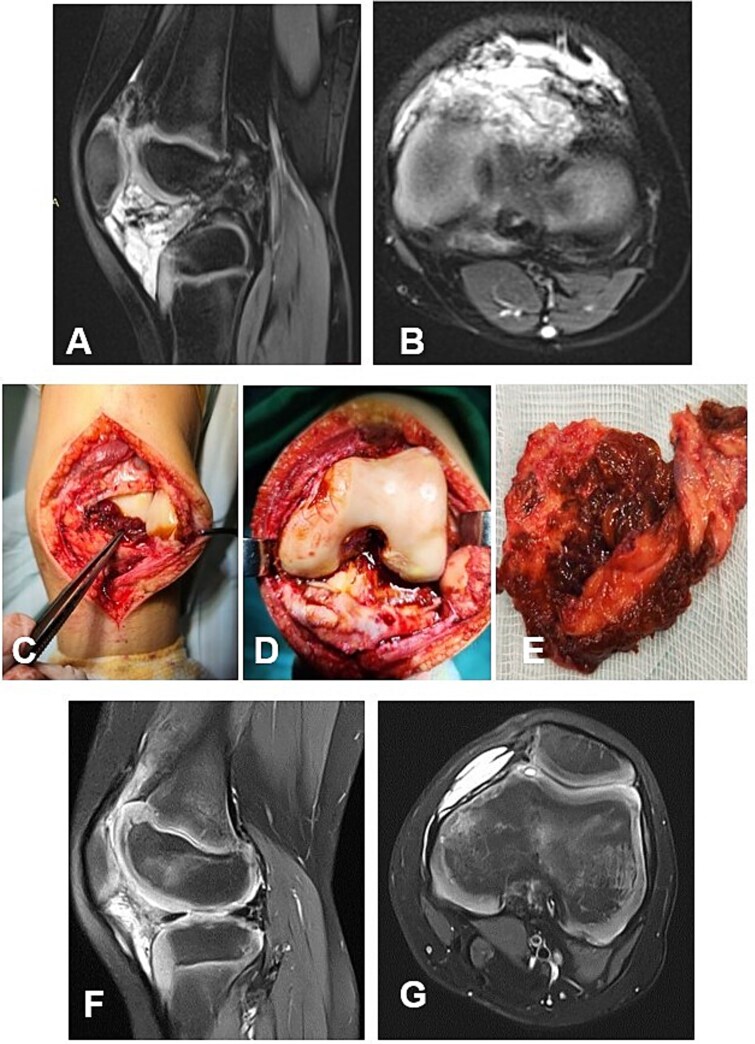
Case 3. (A and B) Preoperative sag and ax MRI TSE PD FS. (C and E) Macroscopic appearance of the pathological synovium before and after its excision. (D) The knee joint following synovectomy. Chondral lesions correspond to Outerbridge grade I. (F and G) Postoperative 12 m. Sag and Ax MRI TSE PD FS—no evidence of recurrence of the resected neoplasm. PD FS, proton density weighting with fat suppression; TSE, turbo spin echo.
